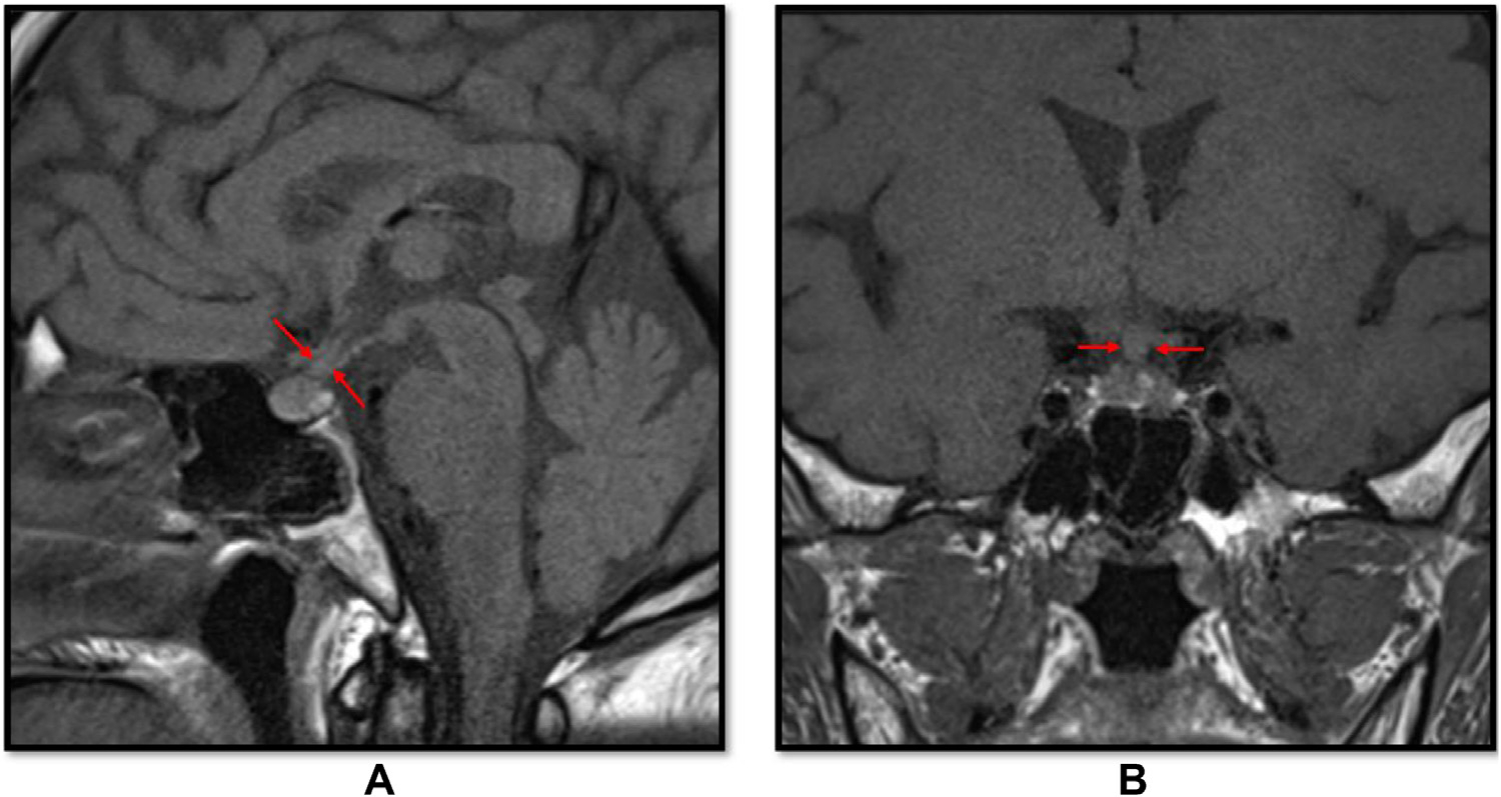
Selected T1 sagittal (A) and coronal (B) images of multiplanar multisequence MRI of pituitary gland without intravenous contrast (avoided due to acute kidney injury), showing mild thickening of the pituitary stalk (red arrows). The width of pituitary stalk measured 4 mm.
